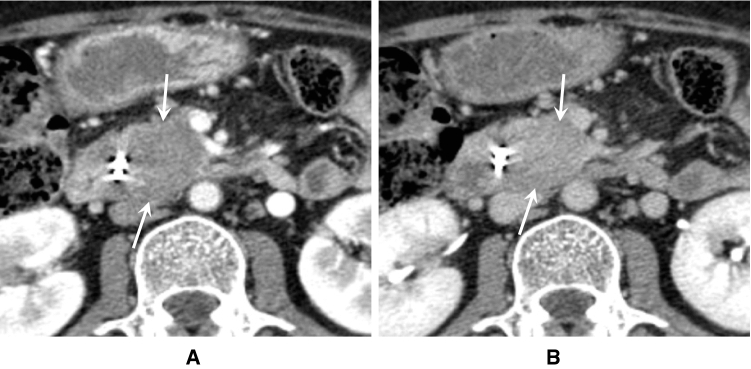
Contrast-enhanced CT images of AIP with focal pancreatic enlargement. a DCE-CT (pancreatic phase) shows a hypovascular lesion in the pancreatic head (arrows). b DCE-CT (delayed phase) depicts a pancreatic head lesion with homogeneous delayed enhancement (arrows)
